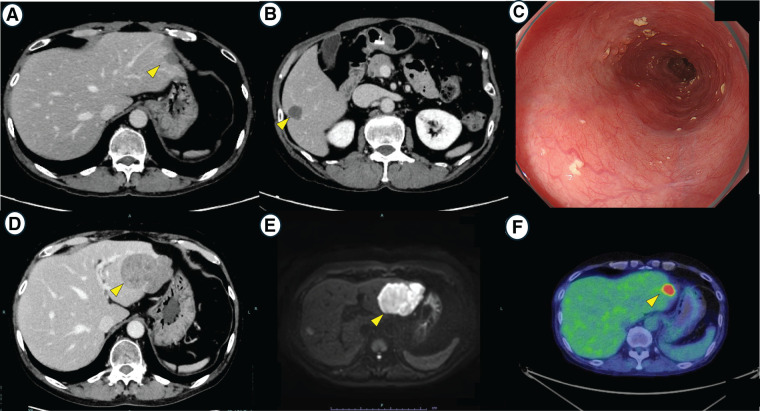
Imaging findings after chemotherapy (A, B) CT imaging after 10 cycles of pembrolizumab plus FP therapy. Contrast-enhanced CT scans demonstrate a marked reduction in the size of multiple hepatic metastases following combined pembrolizumab and 5-FU/cisplatin therapy (arrow). (C–E) Imaging after completion of 20 cycles of S-1 monotherapy. (C) Upper gastrointestinal endoscopy demonstrating complete regression of the esophageal tumor, indicating a clinical complete response. (D) CECT showing that most liver metastases have regressed, except for a residual tumor in the left lateral segment of the liver (arrow). (E) Diffusion-weighted imaging on MRI showing high signal intensity in the residual hepatic lesion (arrow). (F) PET-CT performed approximately 4 months earlier (C–E) revealing intense 18F-fluorodeoxyglucose uptake in the left lateral hepatic lesion (standardized uptake value max 11), consistent with persistent tumor activity (arrow). At the time of imaging, the lesion was small, and the uptake was observed throughout the tumor. FP, fluorouracil + cisplatin; CECT, Contrast-enhanced CT; PET-CT, positron emission tomography-CT
